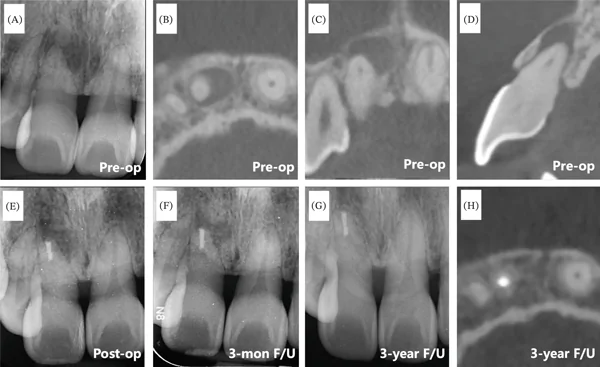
Preoperative and postoperative imaging of Case 2 (Tooth #8). (A) Preoperative PA demonstrating PCO of Tooth #8 with an associated periapical radiolucency. (B–D) Preoperative CBCT images revealing a completely obliterated mid‐root canal, an apical radiolucent lesion, and a reduced intraosseous root length of Tooth #8. (E) Immediate postoperative PA of Tooth #8. (F) Three‐month follow‐up radiograph depicting substantial resolution of the periapical radiolucency. (G,H) Three‐year follow‐up PA and CBCT image confirming complete periapical healing. CBCT, cone beam computed tomography; PA, periapical radiograph.
